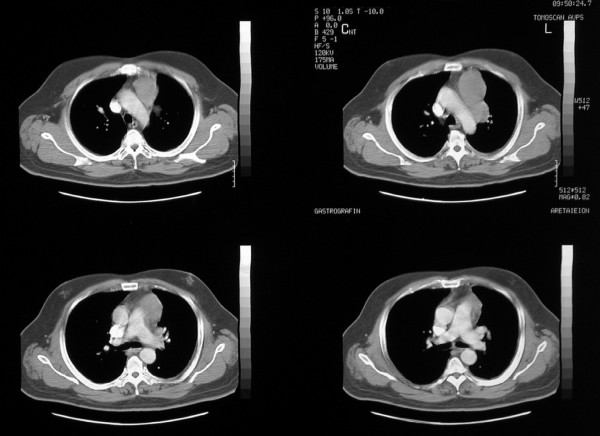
Chest CT-scan revealing the sizeable mediastinal mass in the pre-aortic space extending into the aortopulmonary window.
